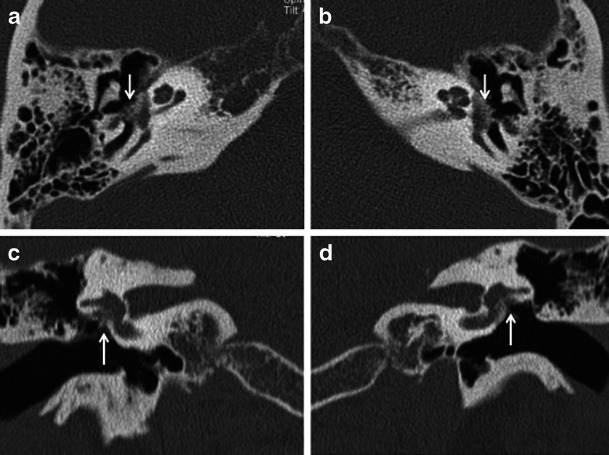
Axial (a,b) and coronal (c,d) HRCT images of the *right* and *left* temporal bone in an adult patient with bilateral severe CHL. Heaped-up bony otosclerotic plaques are noted causing severe bilateral oval window narrowing (*arrows*)
